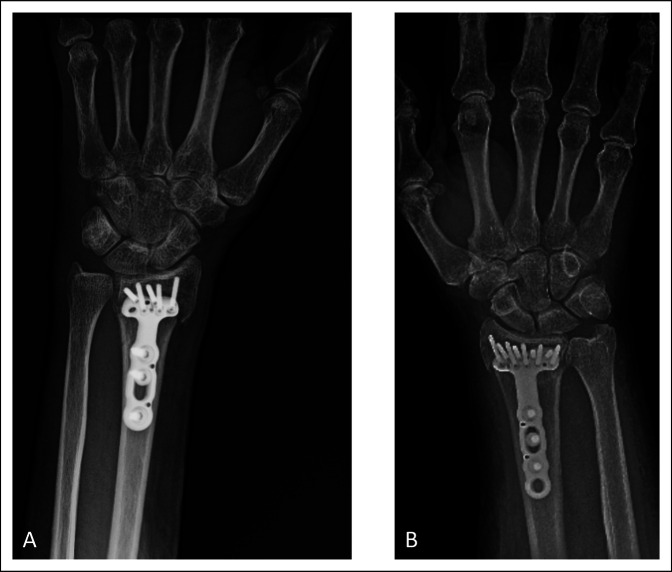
Radiographs showing the distal radius fracture fixation with differing constructs within the same vendor. Both of these fractures were treated with Zimmer Biomet plates. A, This construct had five distal locking pegs that cost $1058.08. Their PRWE baseline score was 96.5 and at 12 weeks was 56.5. B, This construct had nine distal locking pegs that cost $1325.08. Their PRWE score at baseline was 55.5 and decreased to 21.5 at 12 weeks. Both patients presented with AO/OTA 23C type fractures.
